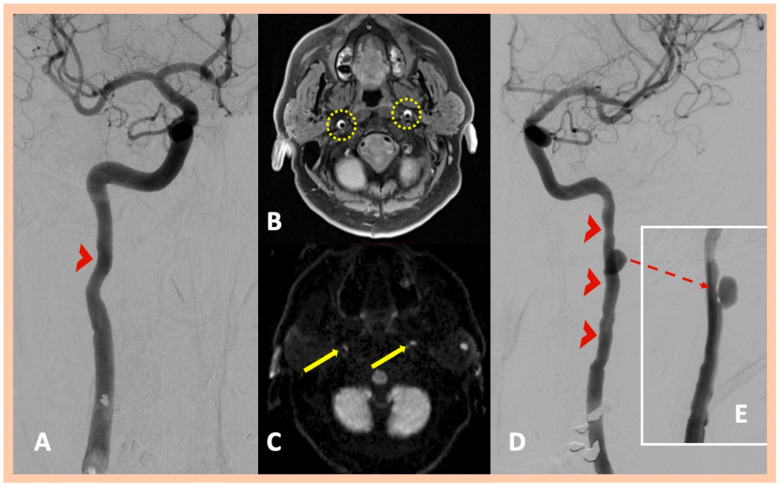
Neuroimaging findings—Patient #3. Three-dimensional fat-saturated black-blood T1-weighted high-resolution (3 Tesla) vessel wall imaging, showing crescent-shaped, hyperintense lesions in the vessel walls of the right and left ICA (Panel (A)), indicating intramural hematoma. Characteristic crescent- or ring-shaped lesions with DWI restriction are detected along the affected segments of the ICA bilaterally (Panel (B)). Digital subtraction angiography showing focal stenosis along the extracranial segment of the right ICA, indicative of intramural hematoma (Panel (C)), and long tapered stenosis along the extracranial left ICA (Panel (D)), with the presence of a dissecting aneurysm (Panel (E)).
